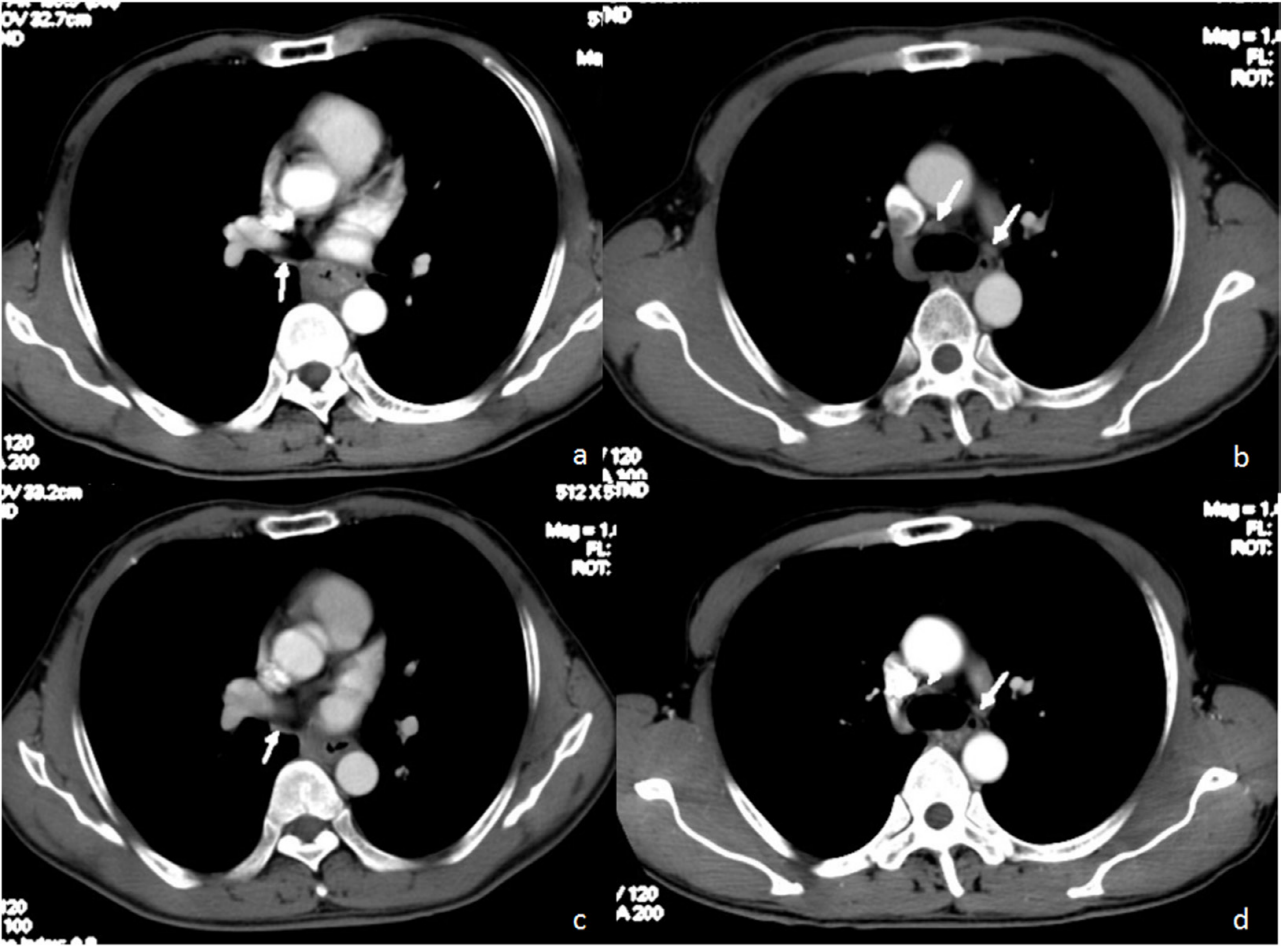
58 year-old man with good prognosis of esophageal squamous cell carcinoma Pre-neoadjuvant chemotherapy CT (a, b) showed MaxLN (arrow): 16x5mm, the number of LN (arrow) was 3. Post-neoadjuvant chemotherapy CT (c, d) showed MaxLN(arrow):13x5mm, the number of LN (arrow) was 3. OS=70.1 months, DFS=70.1 months.
